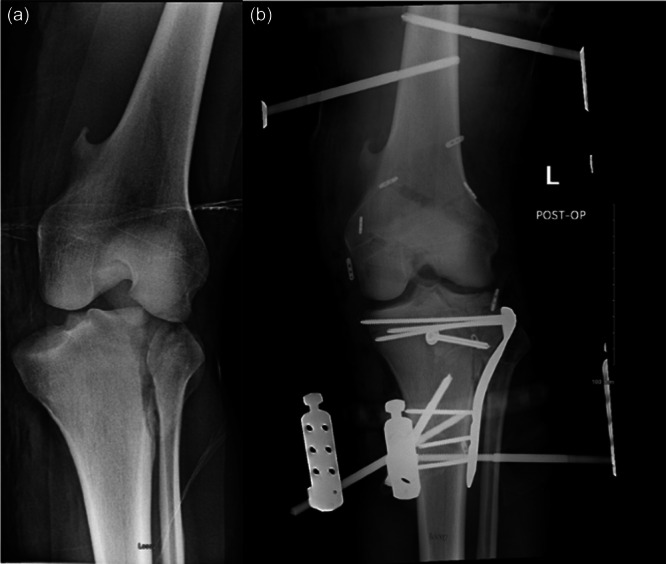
(a) AP radiograph demonstrating lateral tibial plateau split fracture with dislocation of the medial plateau. (B) AP radiograph demonstrating the reduced and stabilized lateral tibial plateau, multiligamentous reconstruction and application of hinged external fixation. AP, anterior–posterior.
